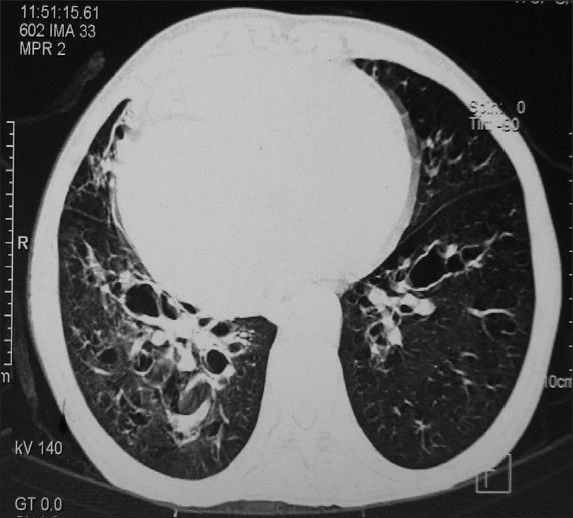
CT chest showing bronchiectasis.
